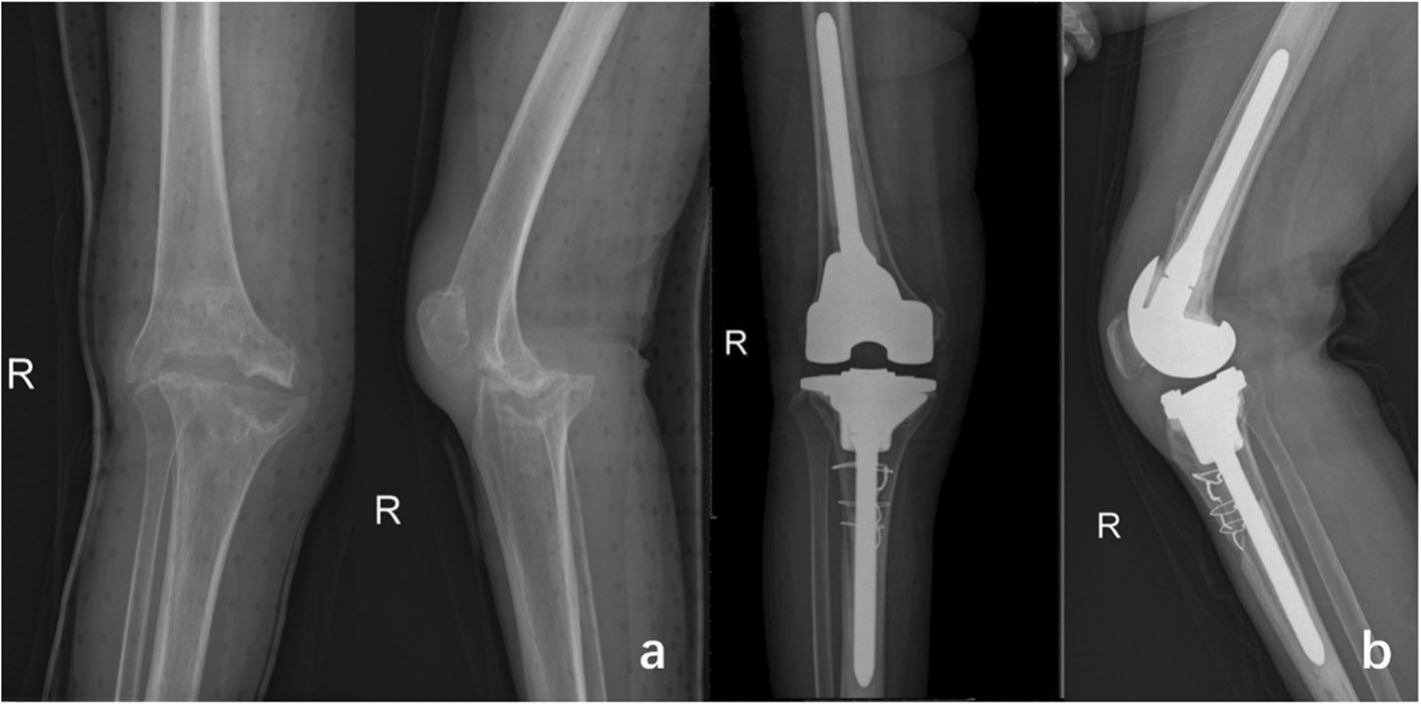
a-After the right knee spacer was removed for 4 months, the X-ray film showed obvious bone defects and reduced space in the distal femur and tibial plateau; b- During the second hospitalization, the knee joint was reconstructed with ACCK prosthesis (ACCK Knee Prosthesis; AK; Beijing, China)
